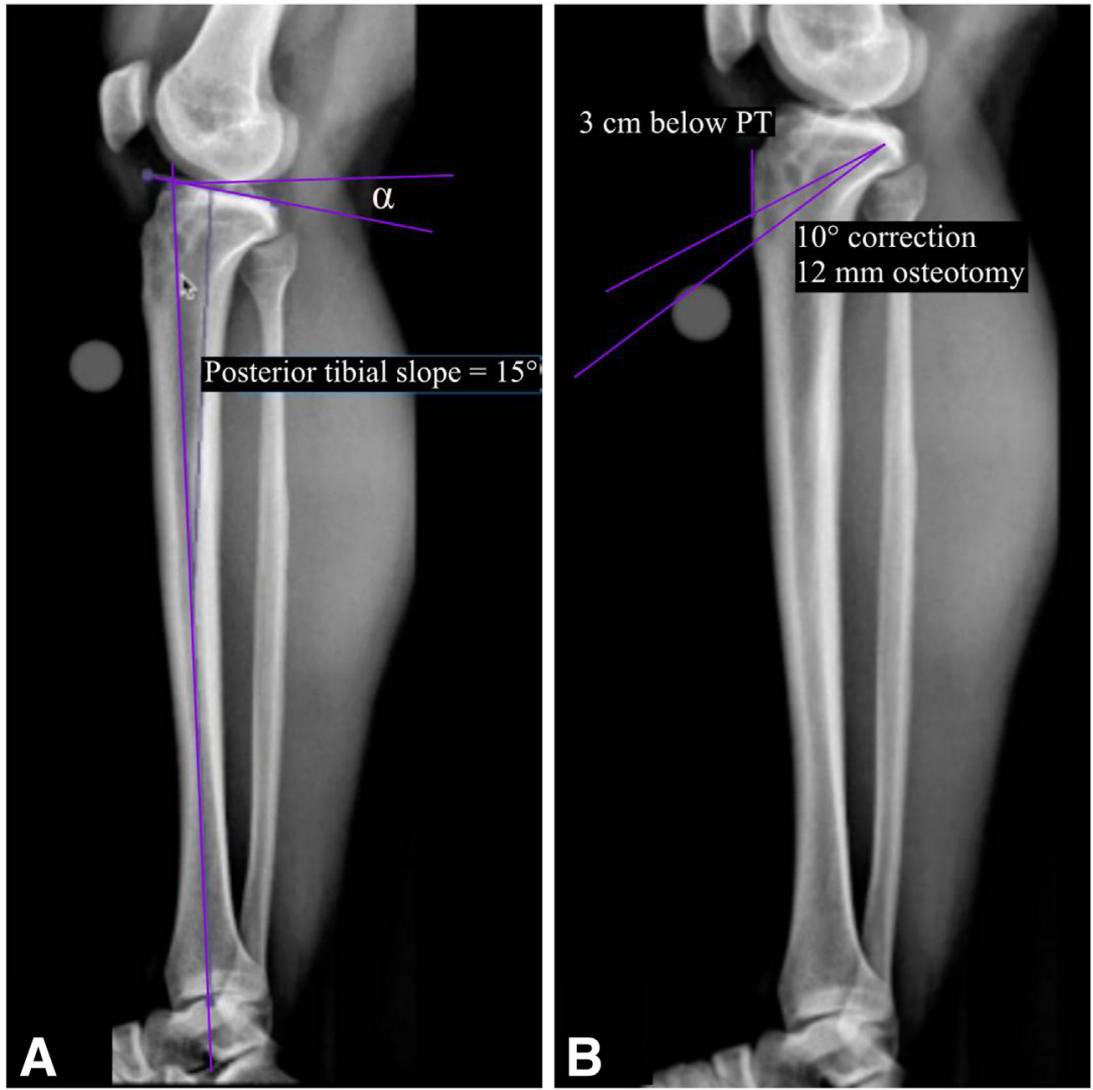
Radiographic measurements (long-leg lateral x-ray views). (A) Measuring posterior tibial slope (α). (B) Determining start point and osteotomy size.
